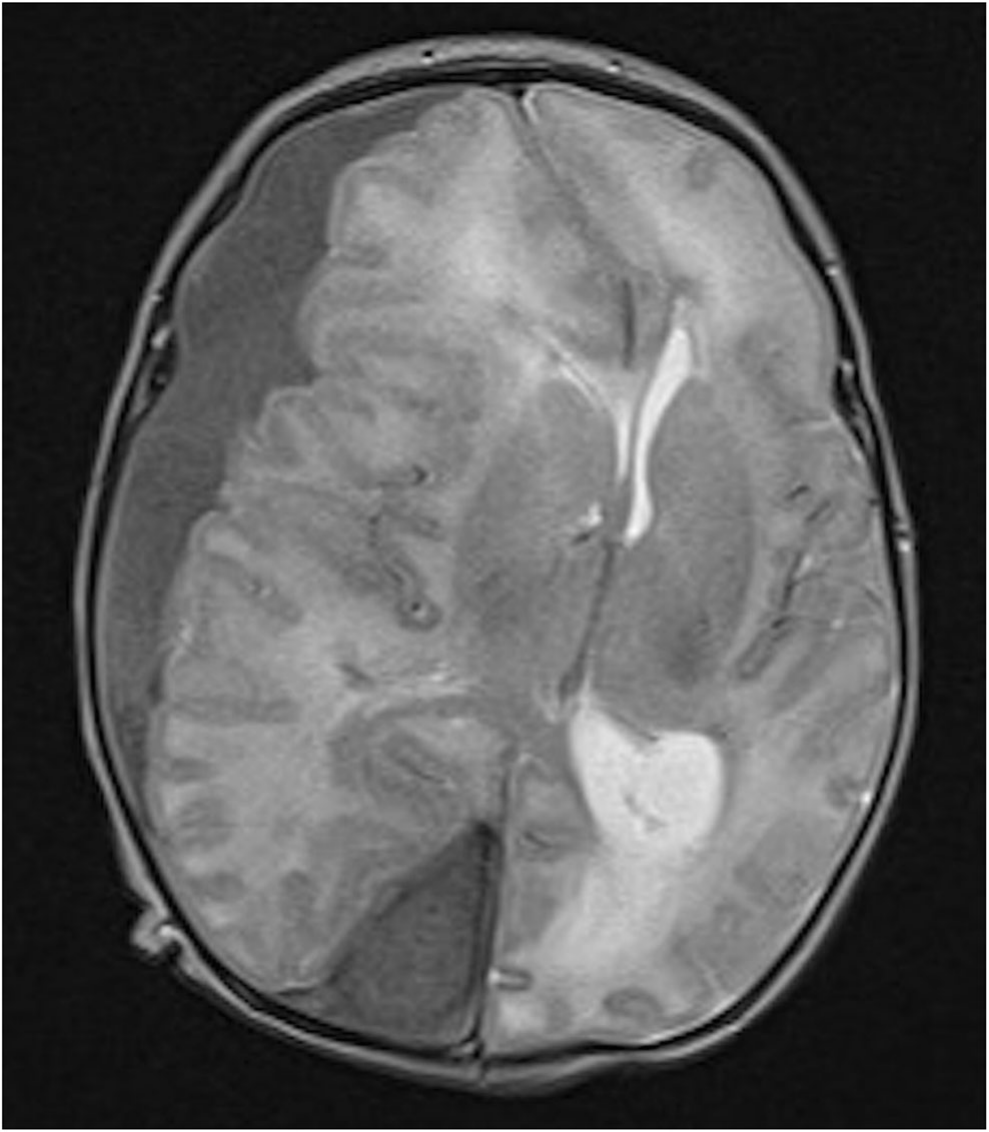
T2-weighted axial MRI. Large right-sided subdural haematoma with severe midline shift. In addition to effacement of the right lateral ventricle, there is enlargement of the trigone of the left lateral ventricle consistent with left-sided hydrocephalus
